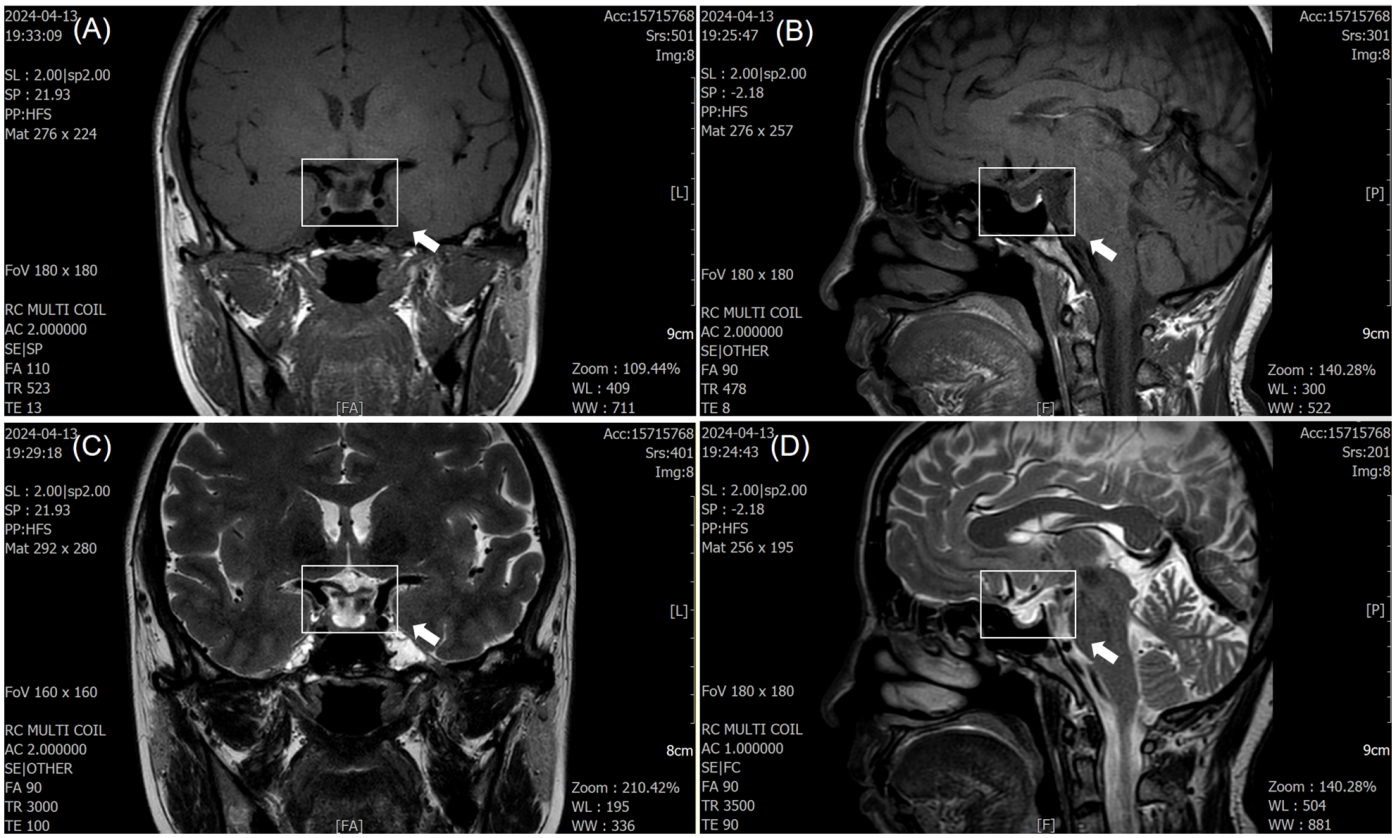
Magnetic resonance imaging (MRI) findings of empty sella syndrome. (A, C) T1- and T2-weighted coronal images demonstrate cerebrospinal fluid (CSF) filling the pituitary fossa (white arrow), consistent with subarachnoid herniation. (B, D) Sagittal images show flattening of the pituitary gland (white arrow), with the pituitary stalk positioned centrally. No optic nerve or cavernous sinus abnormalities were observed.
